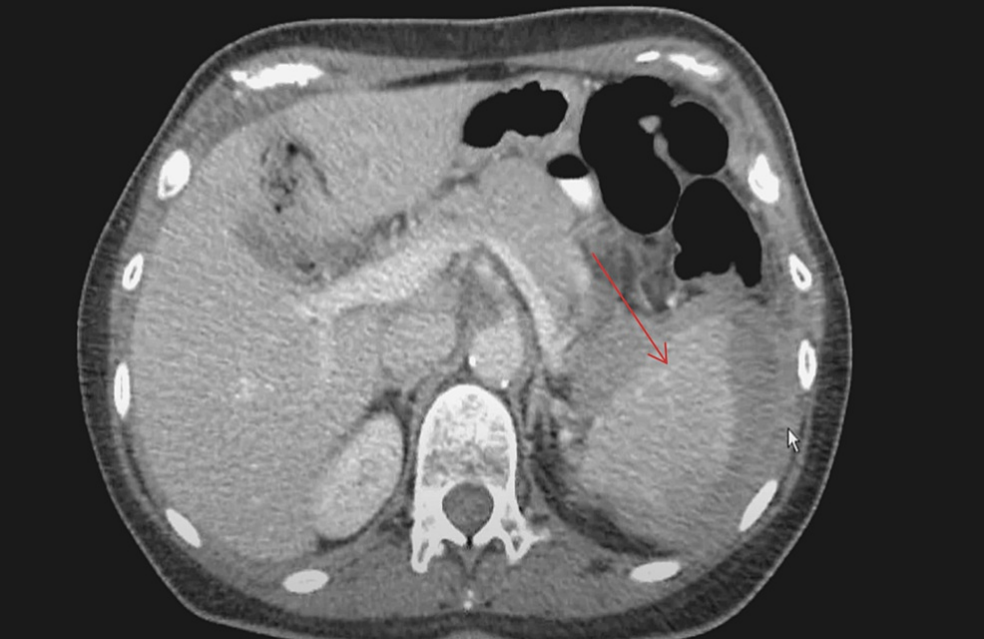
Abdominal CT scan with an IV contrast axial cut showing an irregular contour of the spleen, heterogenous enhancement, splenic parenchyma low-attenuation, and laceration with contrast extravasation (red arrow)
